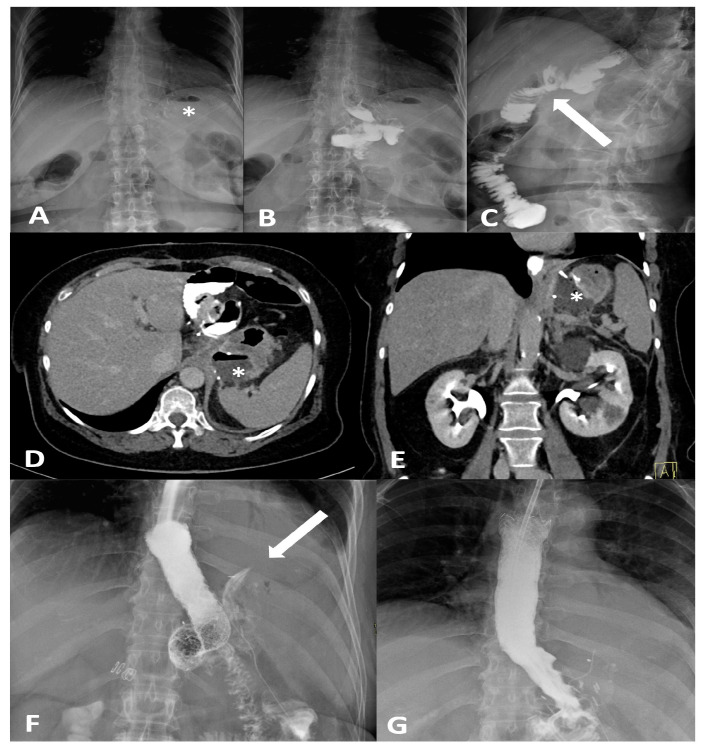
Frontal (A,B) and oblique (C) X-ray for postoperative bariatric surgery assessment. Surgical clips (asterisk) are visible in the epigastric region and a surgical drain is present in the left hypochondrium. The examination was performed following oral administration of water-soluble contrast agent. The esophagus is patent, with normal caliber and course. The gastrojejunostomy (arrow) shows regular opacification. No extraluminal contrast leakage is observed. Opacified small-bowel loops are seen, without evidence of dilatation. Four days later, following the onset of fever and abdominal pain, the patient was re-evaluated with CT. CT equilibrium-phase axial (D) and coronal (E) scans showing postsurgical changes from gastric bypass with creation of an L–L gastrojejunostomy. Between the medial contour of the gastric remnant and the gastric pouch—inseparable from the left diaphragmatic crus, which appears thickened and slightly heterogeneous—a fluid-containing collection (asterisk) is visible. The adjacent adipose tissue demonstrates a heterogeneous appearance, with several small air bubbles present within it. After the placement of an esophageal stent for protection, the follow-up X-ray with oral contrast (F) shows extraluminal leakage of contrast (arrow) originating from the mid portion of the stent, opacifying a retrogastric collection, as also visible on the CT scans. Ten days later, after placement of a nasojejunal tube, the X-ray with oral contrast (G) no longer shows the previously reported extraluminal leakage. The copyright of this figure belongs to the authors.
